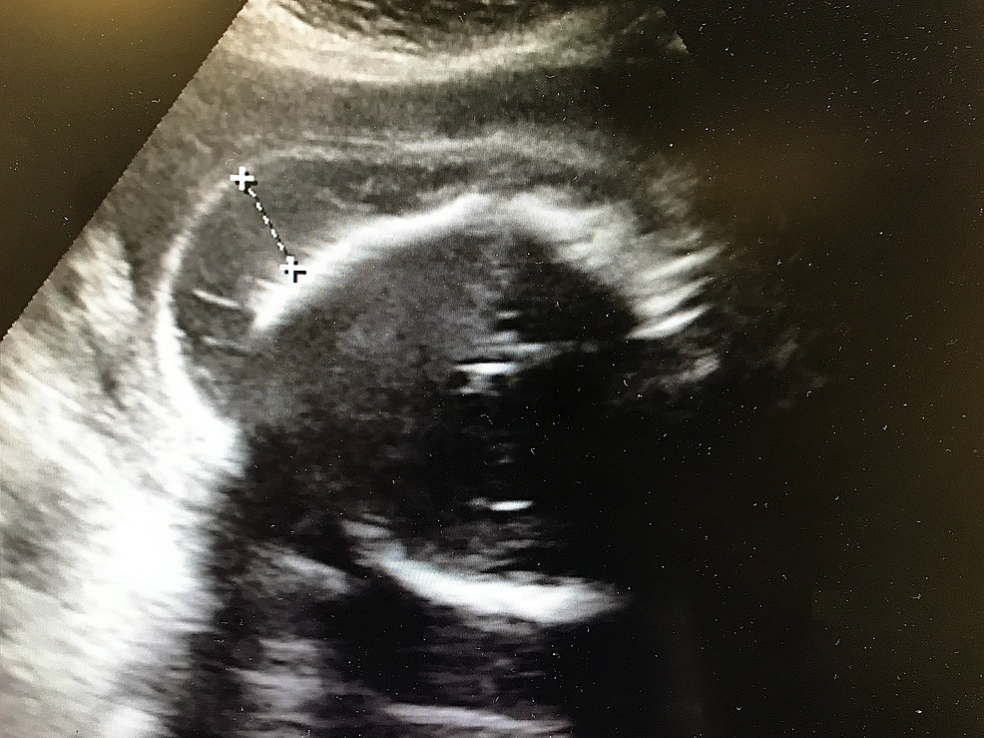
Fetal ultrasound, sagittal view. Posterior aspect of the skull is depicted on the left side of the image.
